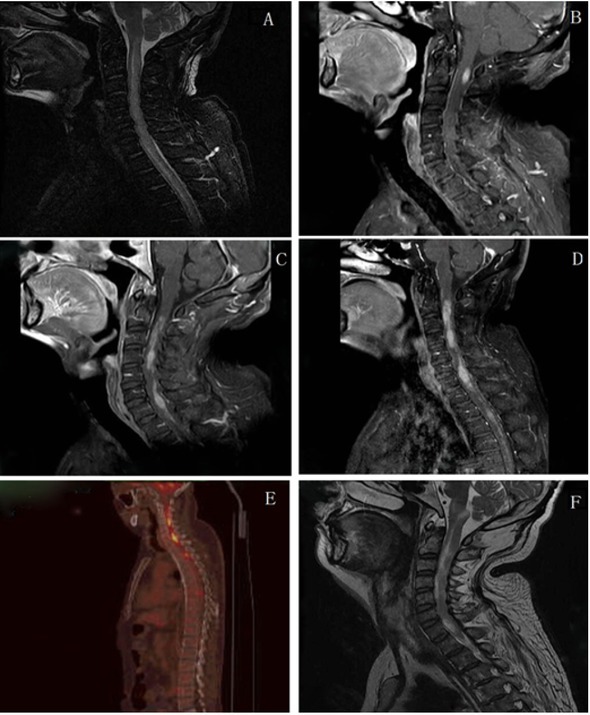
MRI of cervical spinal cord demonstrated multiple lesions with occupied in C2-T1 spinal and brain stem. (March 26^th^) A: Sagittal T2 weighed showed enlargement of the cervical spinal. (April 1^st^) B and C: Sagittal T1 enhanced imaging showed the enhanced lesion in C2-T1 level and brain stem and slightly enlargement of the cervical spinal. (April 29^th^) D: Sagittal T1 enhanced imaging showed multiple abnormal enhanced lesion in C2 below and in the intrathoracic spinal and brain stem, compared with 2016-04-01 images, lesions increased. (May 19^th^) E: Positron emission tomography (PET) showed obvious increased 18-Fludeoxyglucose (18F-FDG) uptake in the C1-C7, T2-T3, T7 spinal cord. (July 20^th^) F: Sagittal T2 showed multiple abnormal enhanced lesion in C2 below and in the intrathoracic spinal and brain stem, compared with 2016-04-29 images, lesions decreased.
